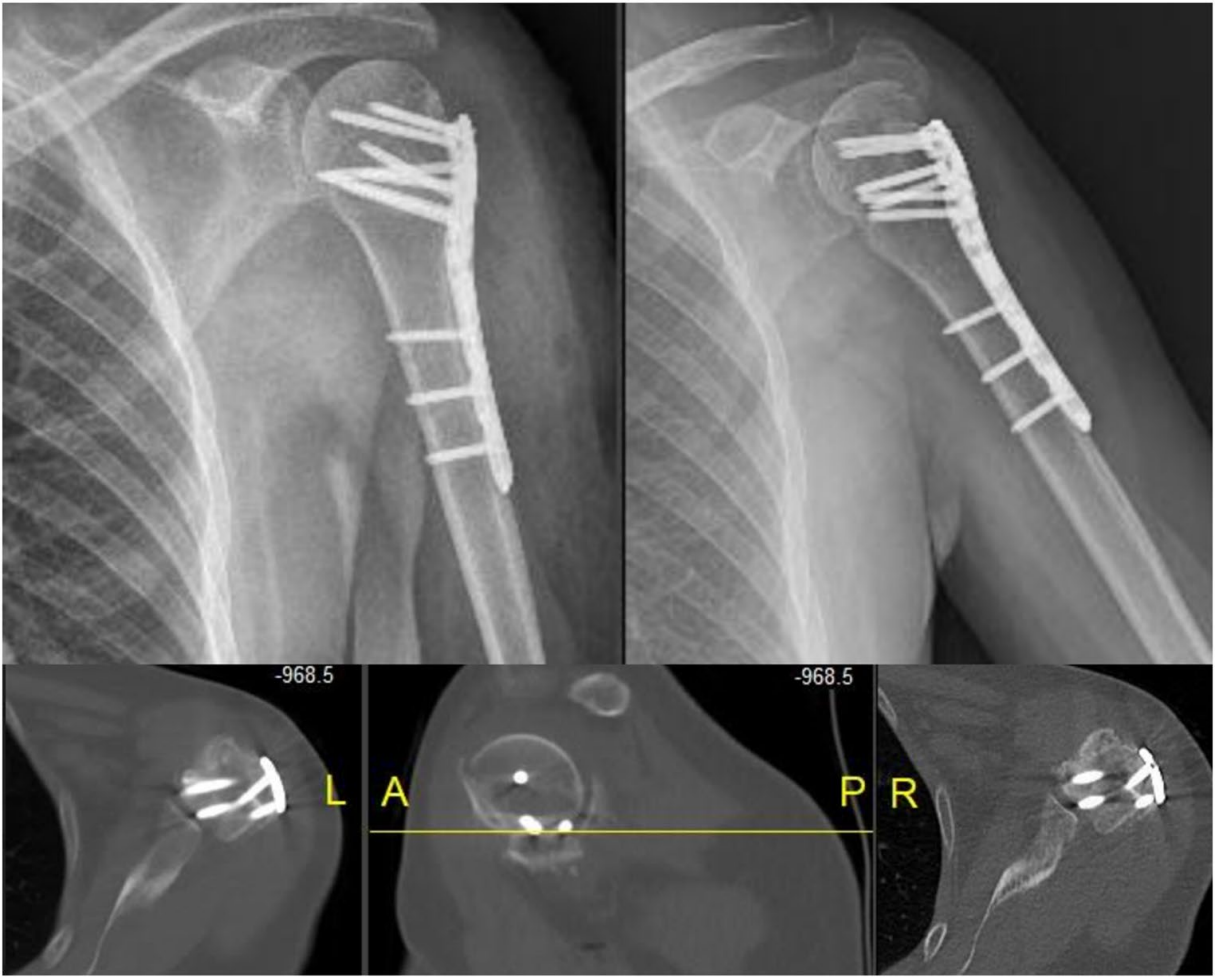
X-ray and CT Imaging of the screw as it extends into the glenoid cavity The screws were revised due to their excessive length and encroachment into the joint line
